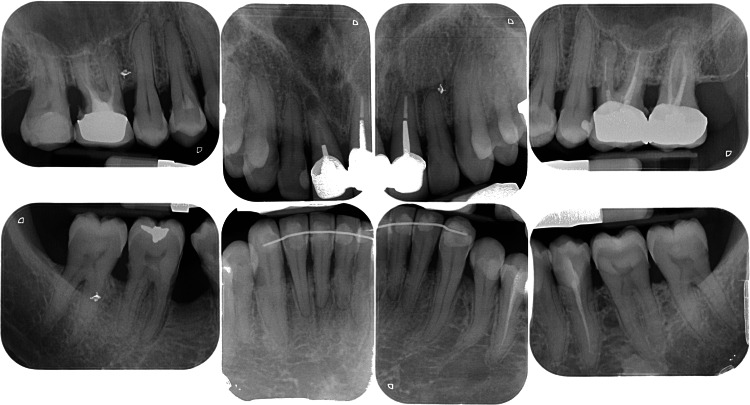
Case 1: initial radiographs. Note the presence of generalized bone loss.
